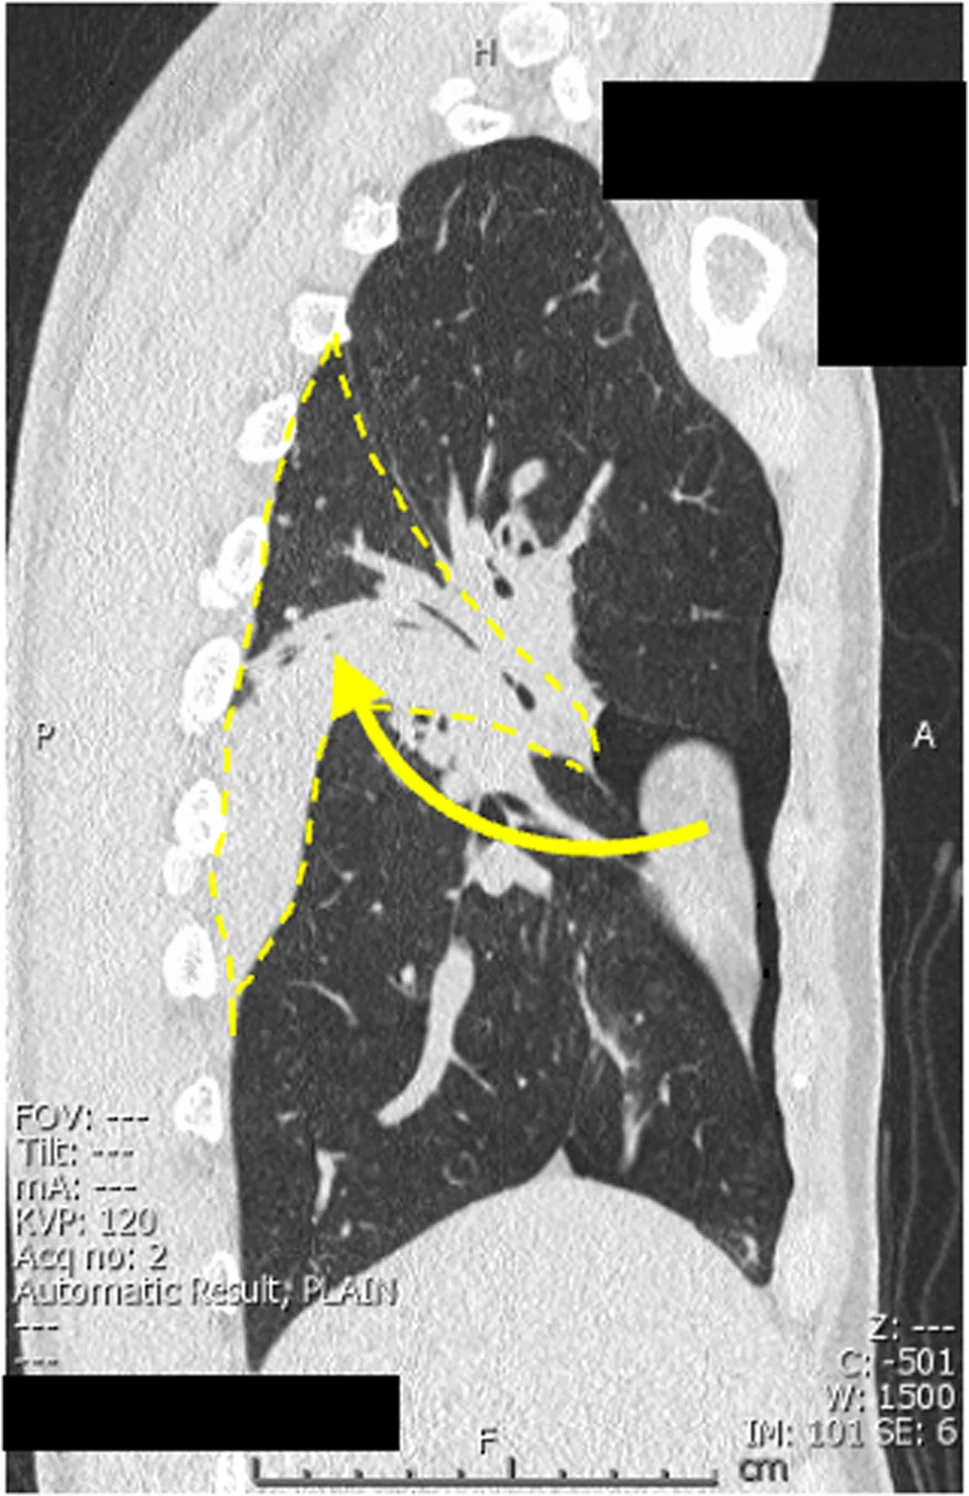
Sagittal chest computed tomography scan showed the right middle lobe with atelectasis, positioned in a dorsal direction (arrow). The outline of the middle lobe is indicated by the dotted line. The right side of the image corresponds to the ventral direction
